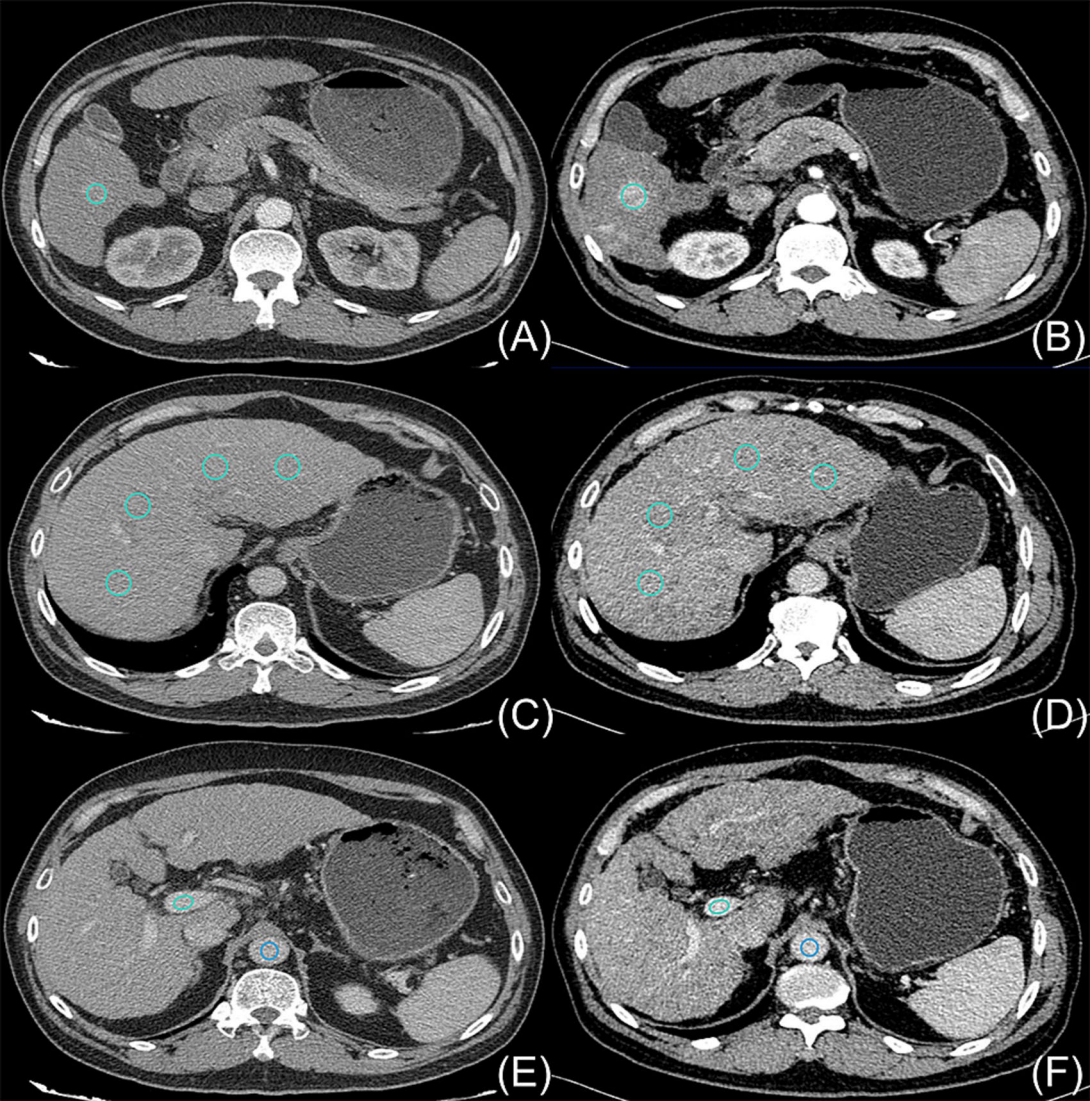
On 24 August 2018, a 54-year-old man with hepatocellular carcinoma underwent initial upper abdominal enhanced CT imaging in which a contrast agent concentration of 300 mg I/mL was used (A,C,E); this patient was subsequently examined on 6 November 2018 using an increased contrast agent concentration of 350 mg I/mL (B,D,F). The light blue circles indicate the ROIs outlining the hepatocellular carcinoma parenchyma (A,B), the liver parenchyma (C,D), and the portal vein (E,F). The dark blue circles in (E,F) indicate the ROIs outlining the aorta.
